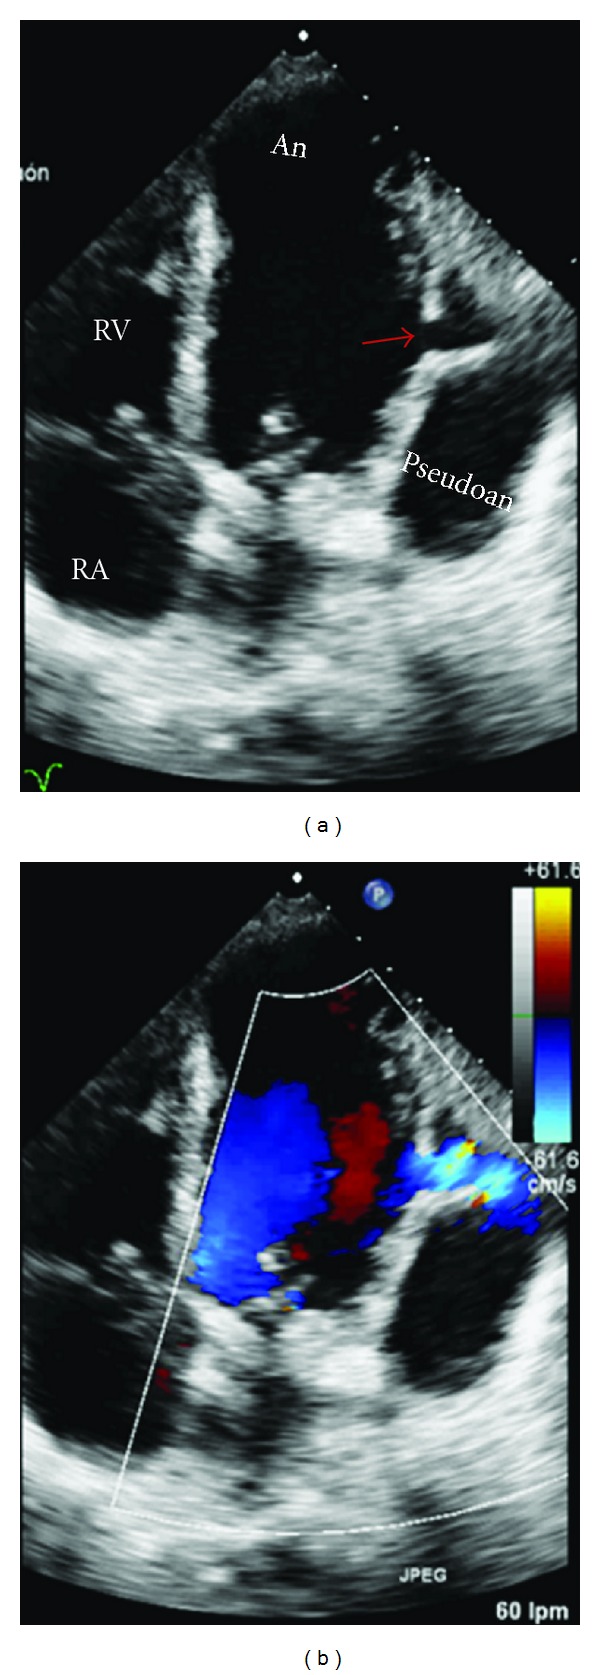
Two-dimensional (a), and color Doppler four-chamber view (b) images showing an apical aneurysm (LVAn) and lateral pseudoaneurysm formation (red arrow) with shunt from the left ventricle to the pericardial space. RV-right ventricle, RA-right atrium, Pseudoan-pseudoaneurysm.
